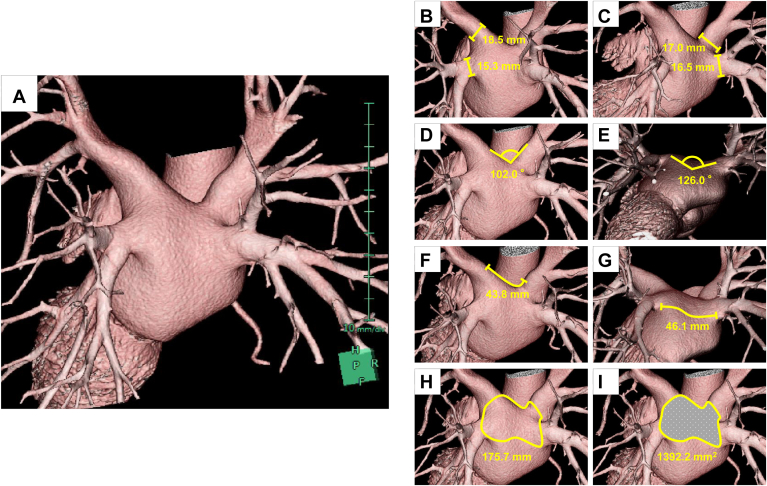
Representative preprocedural CCTA findings in a patient with PAF who had no recurrence of ATA after CB-PVI, demonstrating a short LAPC (<199.6 mm). A: Reference axial slice. B: Ostial diameters of the LSPV and LIPV. C: Ostial diameters of the RSPV and RIPV. D: LA roof angle. E: LA bottom angle. F: LA roofline length. G: LA bottom line length. H: LAPC. I: LAPA. ATA = atrial tachyarrhythmia; CB-PVI = cryoballoon-based pulmonary vein isolation; CCTA = cardiac computed tomography angiography; LA = left atrial; LAPA = left atrial posterior wall area; LAPC = left atrial posterior wall circumference; LIPV = left inferior pulmonary vein; LSPV = left superior pulmonary vein; PAF = paroxysmal atrial fibrillation; RIPV = right inferior pulmonary vein; RSPV = right superior pulmonary vein.
